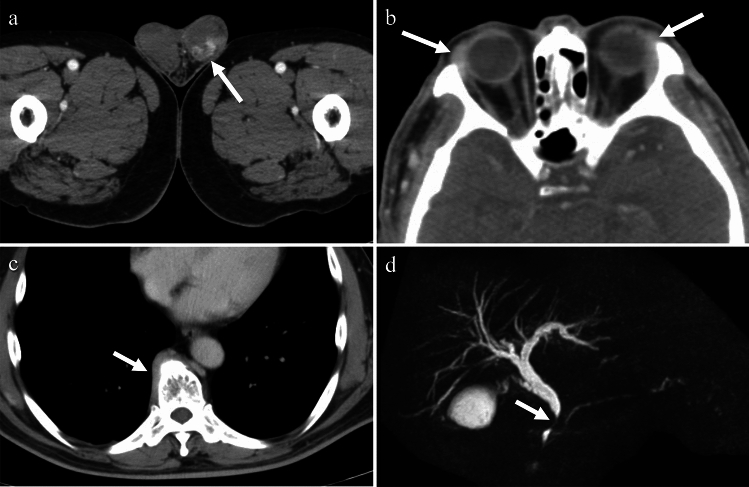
Man in his 60 s with IgG4-related disease presenting with left groin pain. a On contrast-enhanced CT, marked enhancement of the left epididymis (arrow) is accompanied by thickening of the scrotal wall, indicating epididymitis. b Enlargement of both lacrimal glands (arrows) is seen on contrast-enhanced CT. c Excessive soft tissue around the thoracic vertebrae (arrow) is shown on contrast-enhanced CT. d Lower bile duct stenosis (arrow) was observed on magnetic resonance cholangiopancreatography. These indicate abnormalities in various sites consistent with IgG4-related disease
